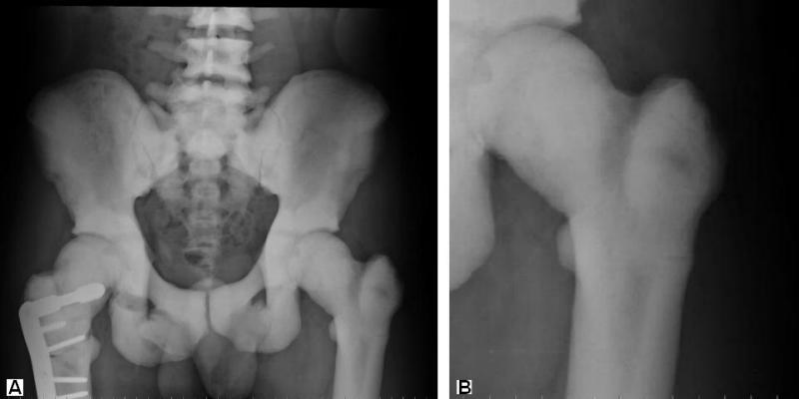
(a) Anteroposterior radiograph of the pelvis obtained 10 weeks after initial fixation. Note the lack of healing progression of the right femoral fracture and the stress fracture now visible in the left femur and better demonstrated in (b).
